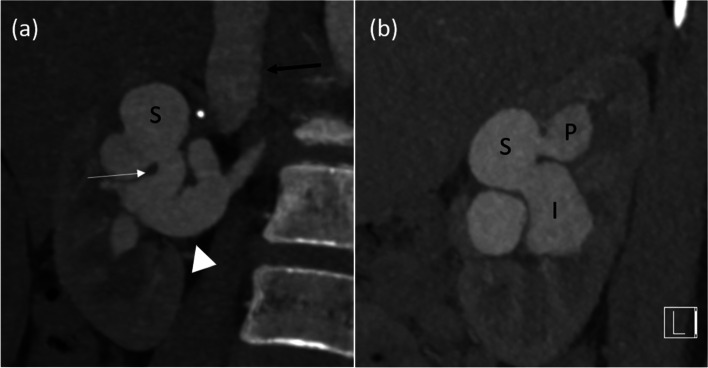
Selected maximum intensity projection arterial phase contrast enhanced CT images performed at diagnosis. a Coronal reformatted image showed an aneurysmal AVF at right renal hilum. The fistula (white arrow) connects an aneurysmally dilated anterior division of right renal artery (white arrowhead) with the superior venous varix (marked with “S”). Early opacification of IVC detected on arterial phase, indicating high flow shunting. b Sagittal reformatted image demonstrated the three interconnecting venous varices – superior (marked as “S”), inferior (marked as “I”) and posterior (marked as “P”)
